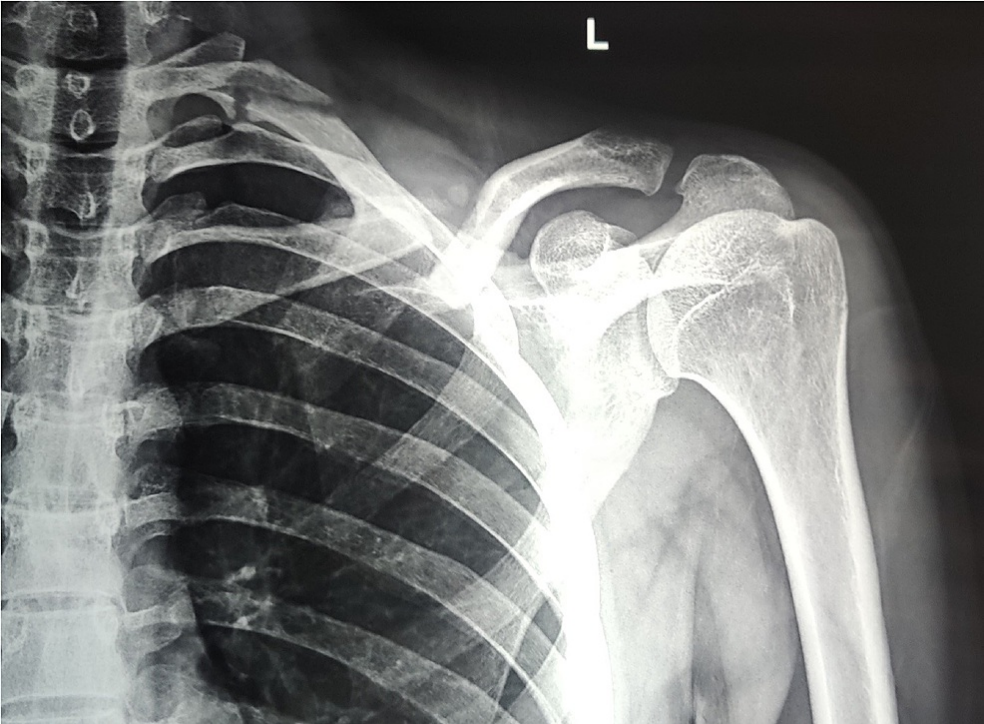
Radiograph showing left clavicle non-union in a patient managed conservatively
